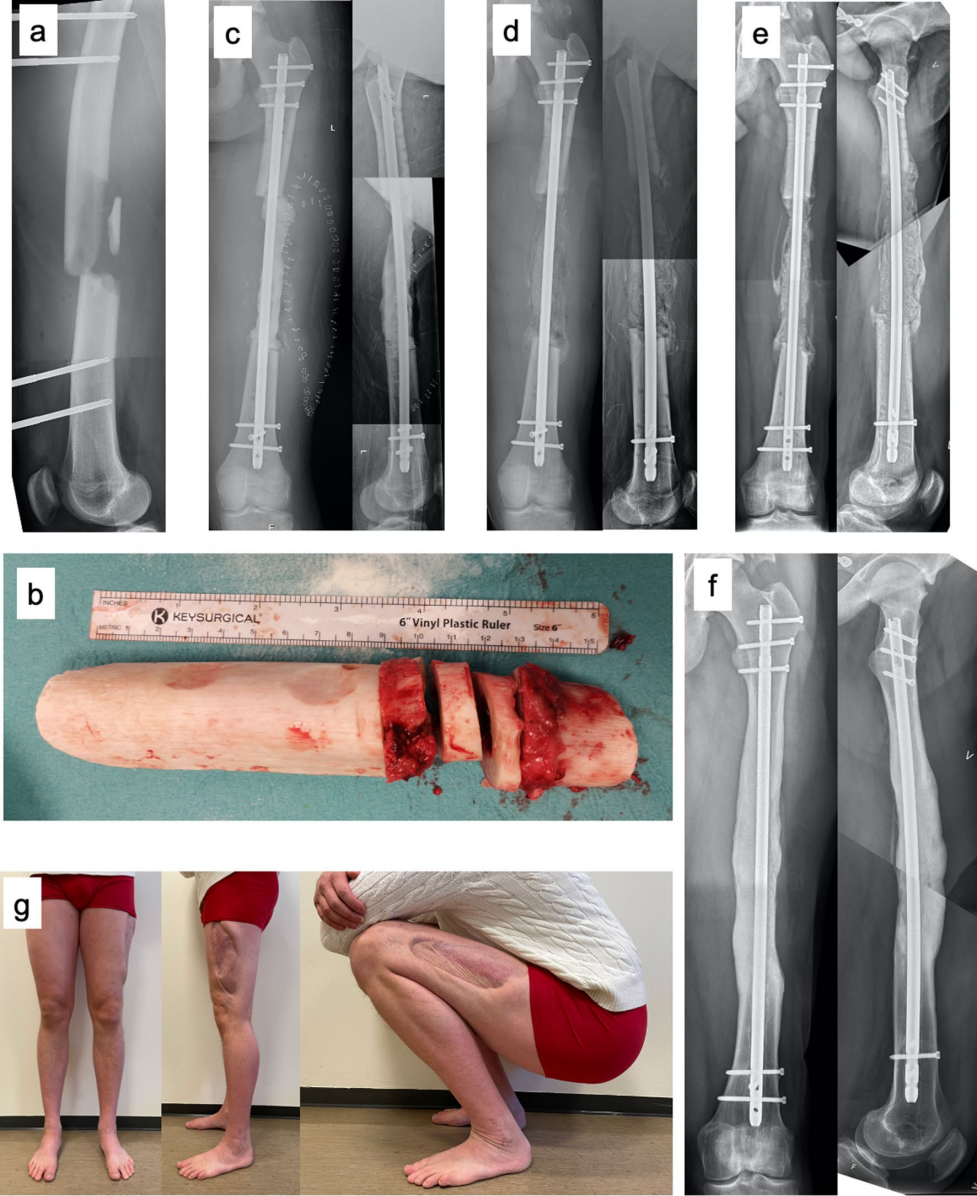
17-year-old male who sustained a Gustilo–Anderson IIIB open femoral fracture and an open book pelvic injury in a motorcycle accident. After initial external fixation (a), the femoral fracture was stabilized with a retrograde intramedullary nail. However, the patient developed a fulminant infection leading to removal of the nail and repeated external fixation. He had positive wound cultures for E. coli, Bacteroides, Clostridia and Staphylococcus capitis and was treated with antibiotics (Penicillin, Ciproxin, Metronidazole and Linezolid) in addition to repeated wound irrigation and debridement. Eventually, 15 cm of devitalized femur had to be removed (b). After 11 surgical procedures on the femur and 30 days after the initial injury, the femur was stabilized with an antegrade intramedullary nail, and the bone void was filled with a gentamicin loaded PMAA cement spacer (c). 31 days later, IMT stage 2 was performed, and the bone void was filled with bilateral iliac crest autograft, blended with adjuvant allograft (d). Radiographs taken 6 weeks postoperatively show incipient ossification of the graft (e). At follow-up 24 months later, the patient presented with a good functional result and the radiographs showed good ossification of the graft (f, g)
